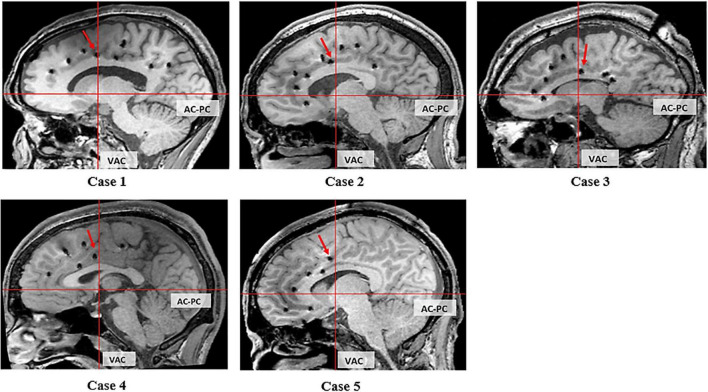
Sagittal T1 MRI of 5 cases. The red arrow indicates the electrode evoking grasping/groping.
